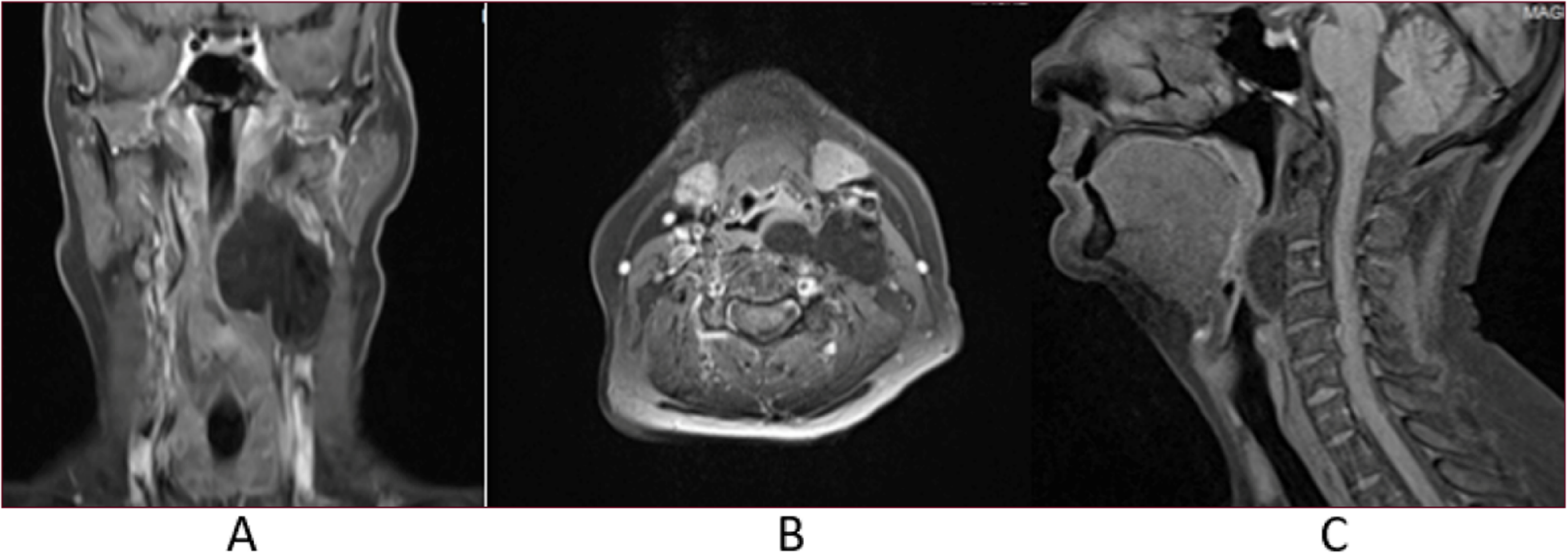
MRI T1-weighted with fat suppression coronal (a), axial (b), and sagittal (c) images depicting a homogeneous loss of signal within the lesion
